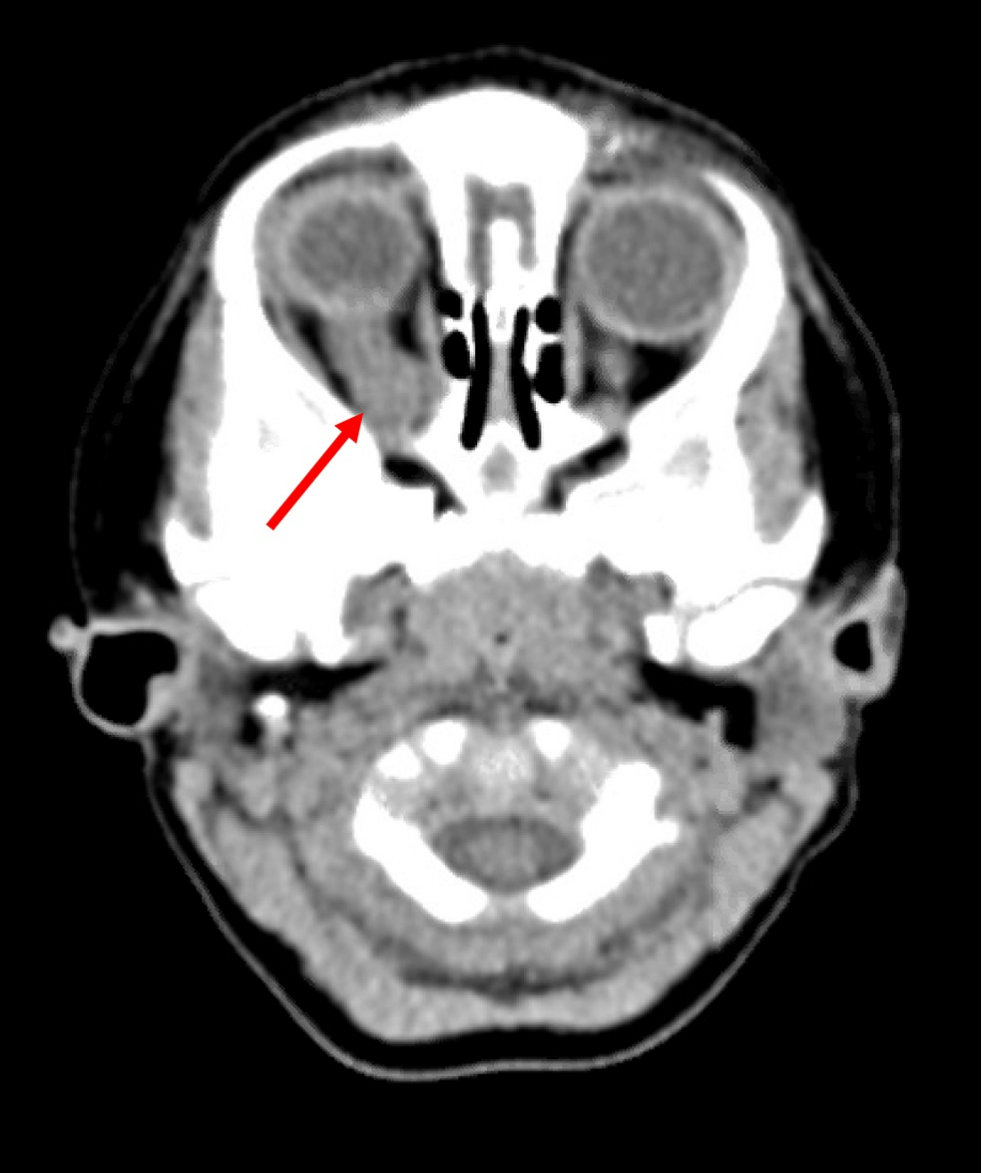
Noncontrasted axial CT of the brain with images centered through the optic nerve demonstrating diffuse thickening with mass-like appearance of the right optic nerve. There is also a thickening and mass-like appearance of the left optic nerve.
